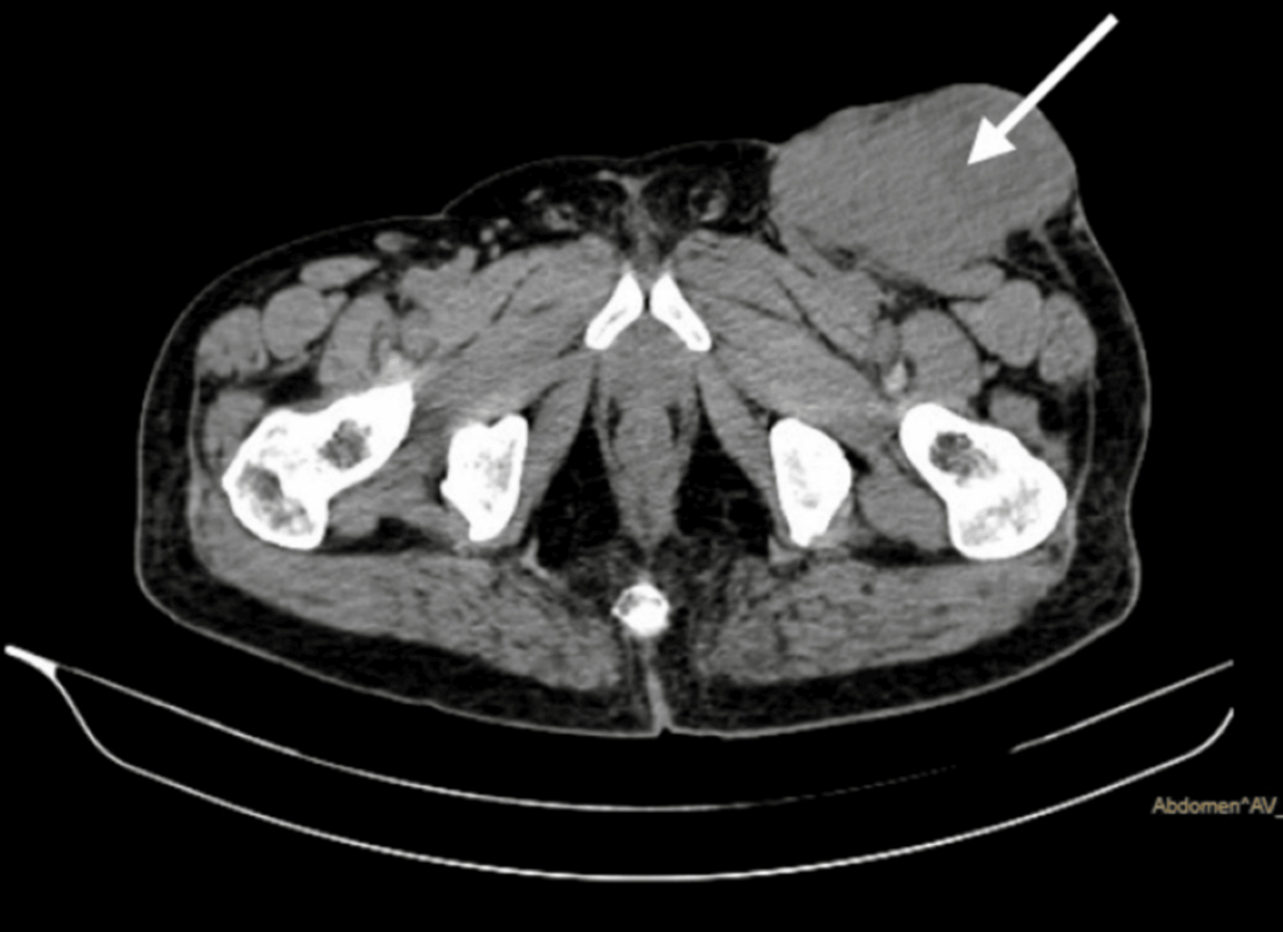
Contrast-enhanced computed tomography - pelvis shows a well-defined hyperdense soft tissue lesion (white arrow) in the left inguinal region.
